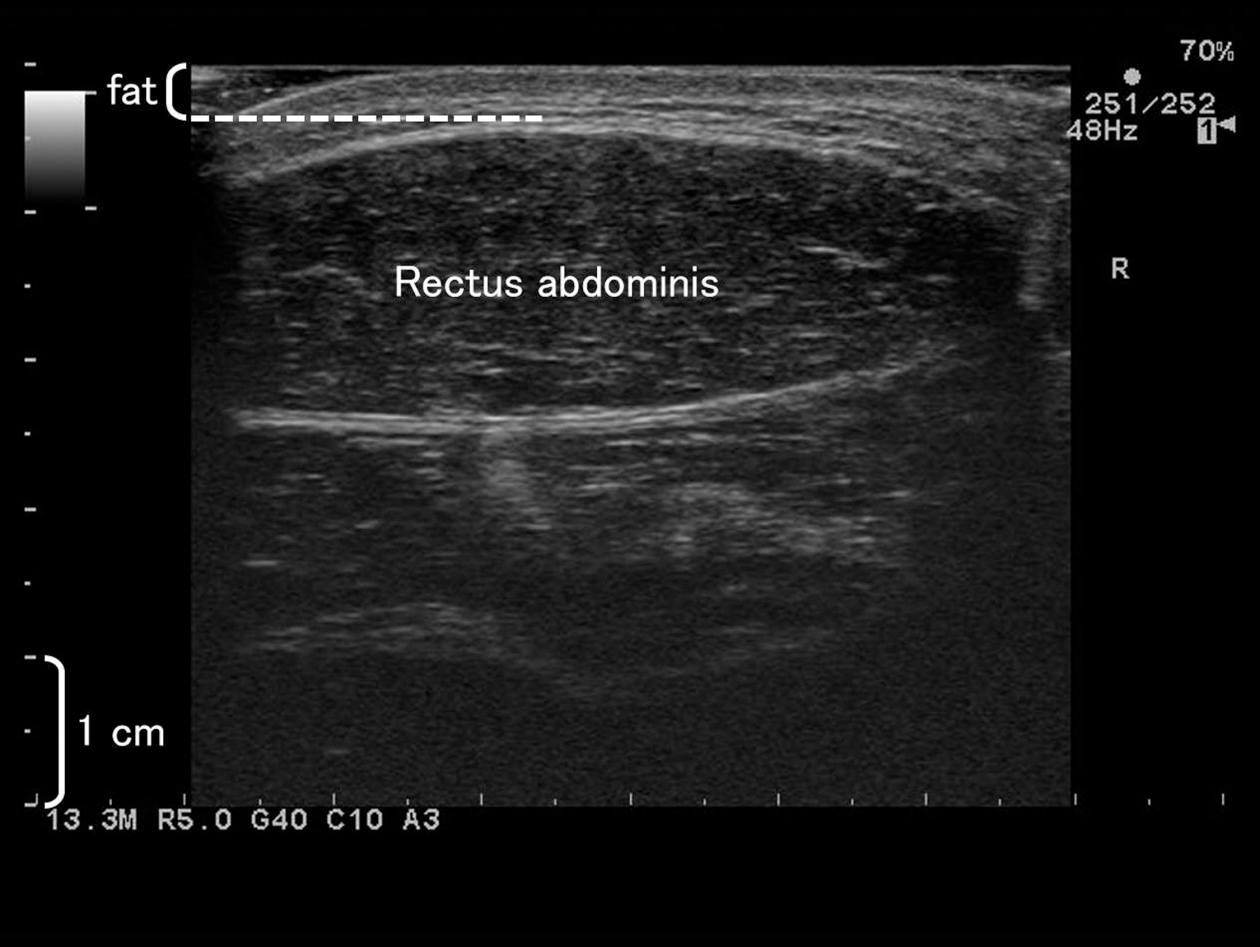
A typical ultrasound image of the abdomen. The white broken line shows the border between subcutaneous fat and the rectus abdominis.
